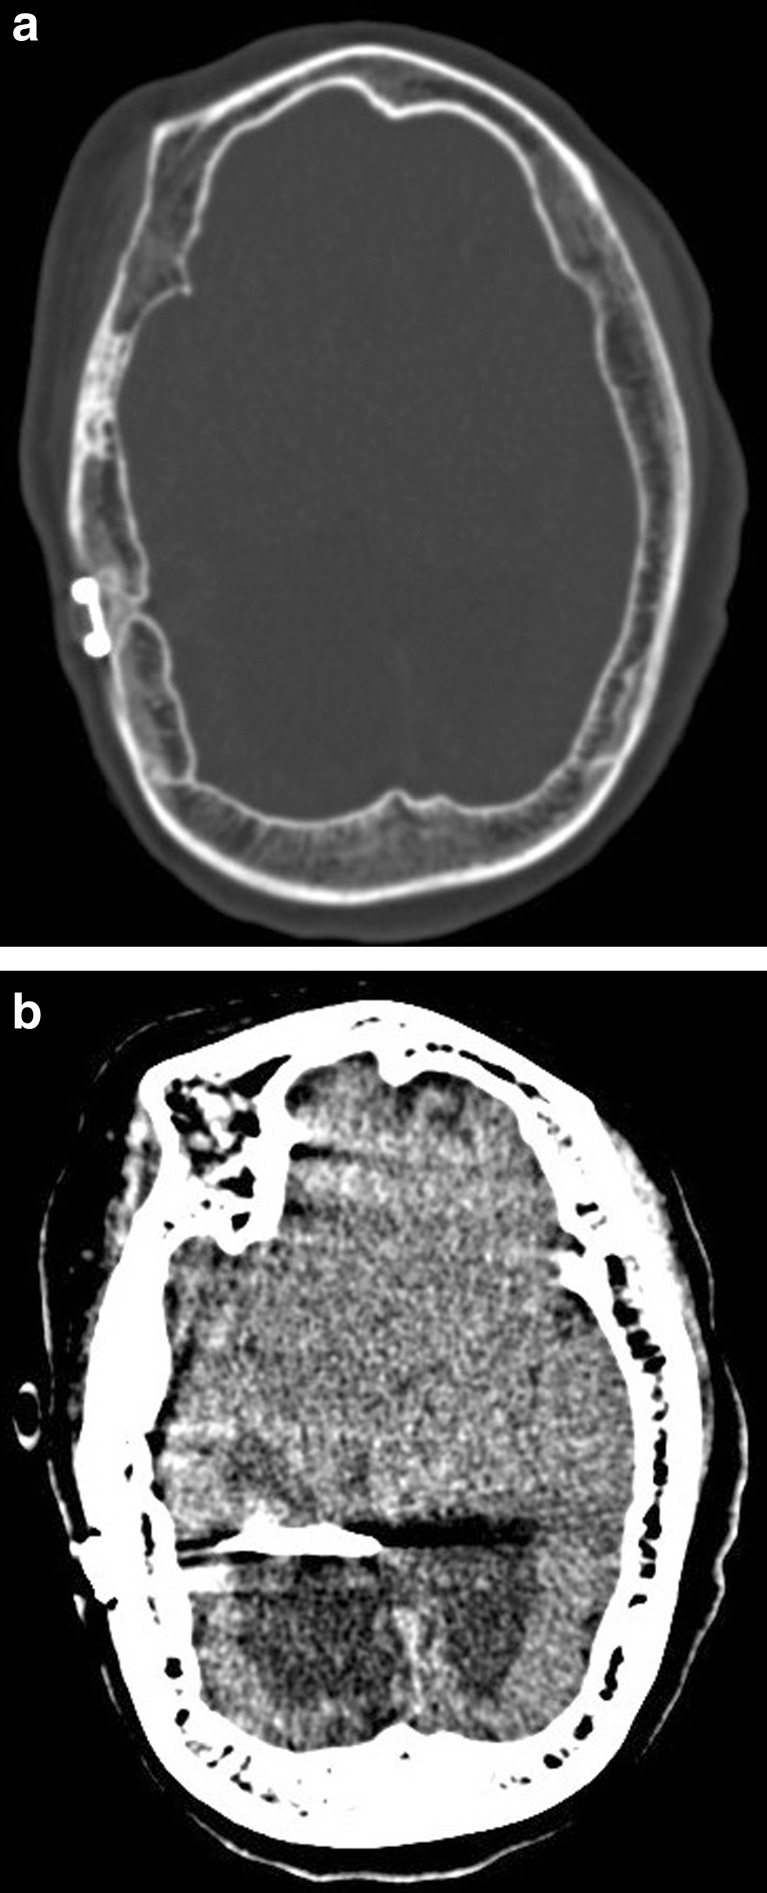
CT scan in bone and brain setting showing a bilateral calcified subdural mass with a thin hypodense area between the inner and outer rims
